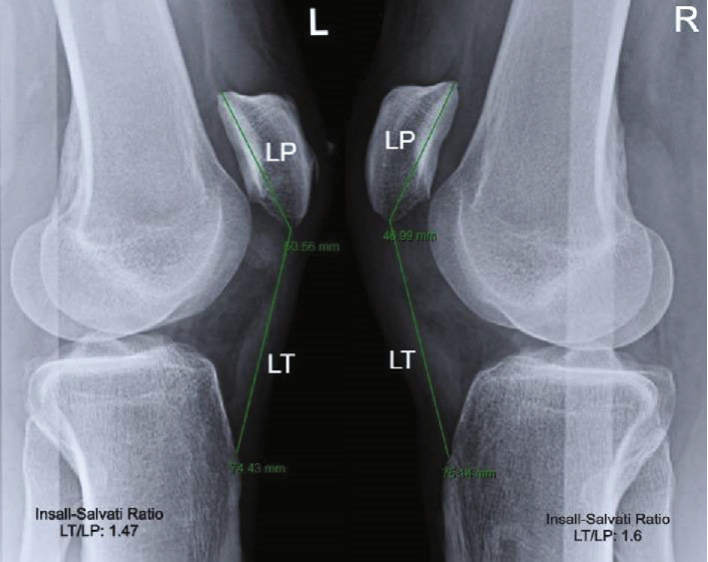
Lateral knee radiographs after bilateral patellar tendon rupture, showing patella alta, knee effusions, and irregularity of the patellar tendons. Confirmed diagnosis using the Insall-Salvati ratio.
